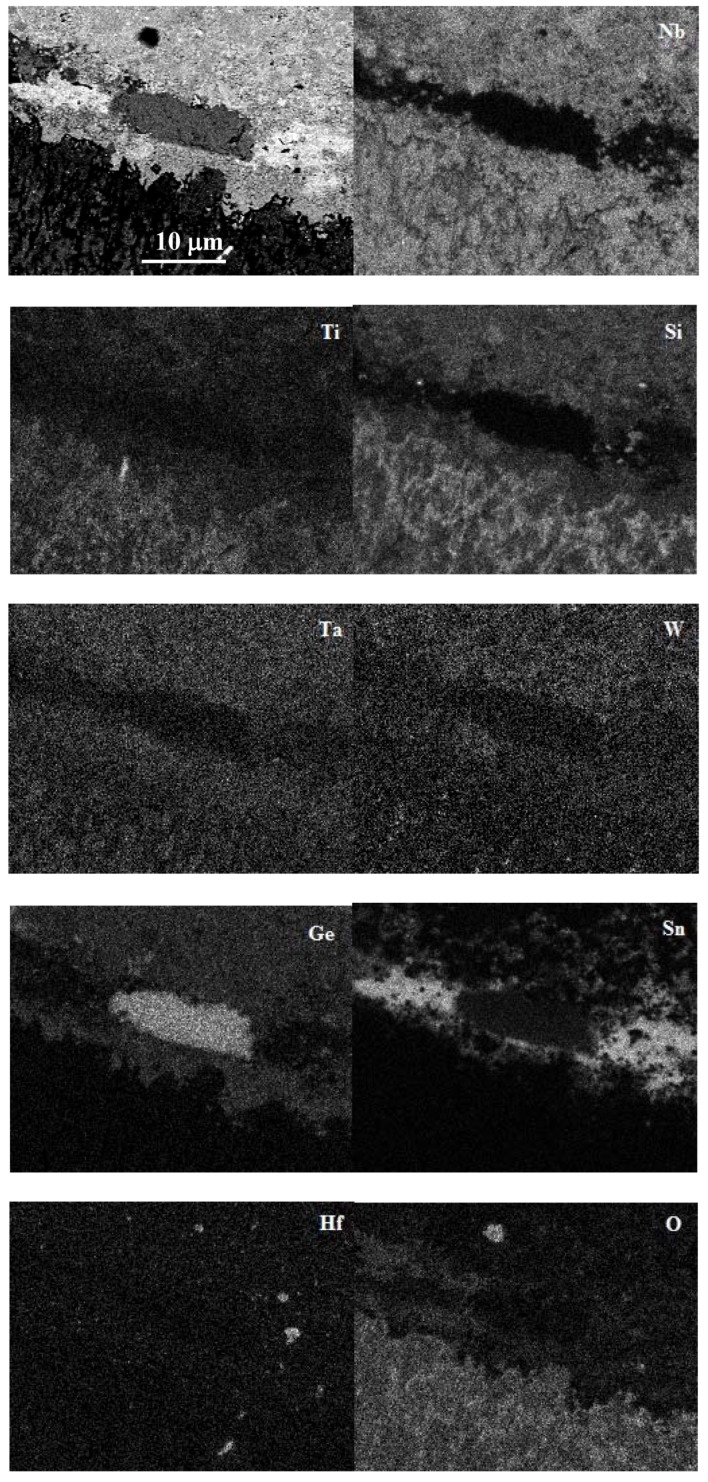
BSE image and X-ray maps of a region in diffusion zone 1.
